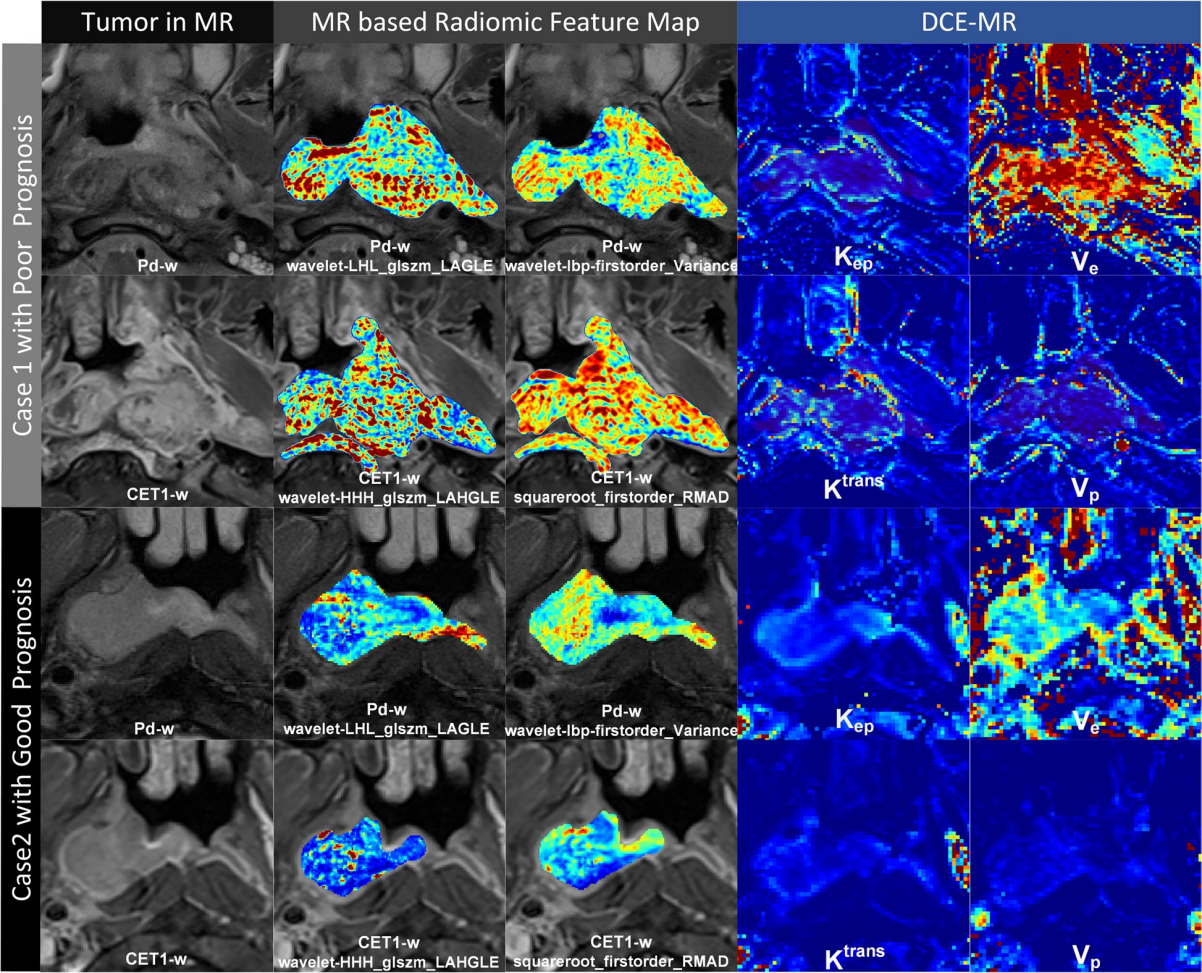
Typical examples of poor prognosis and good prognosis. Each case provides 10 subgraphs, including 2 MR images, 4 radiomics feature maps and 4 DCE-MR parameter images. The name of each subgraph is marked below itself
